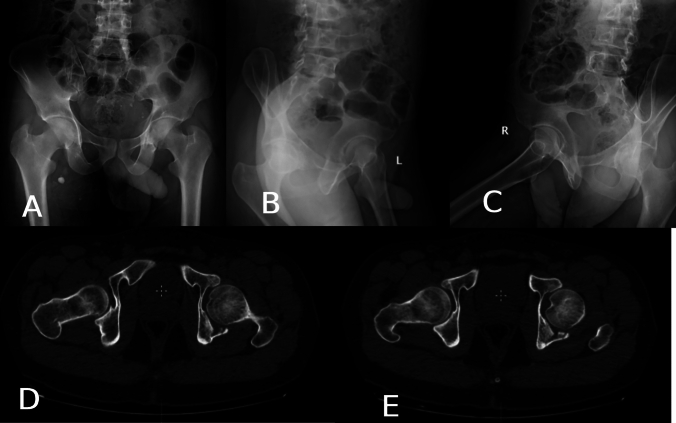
Pure impaction injury; A AP view, B Iliac oblique view, and C Obturator oblique view showing no detectable fracture lines in the left hip, D, and E, axial CT cuts showing large, impacted area of the articular surface of the posterior column with incomplete fracture lines not reaching the surface
